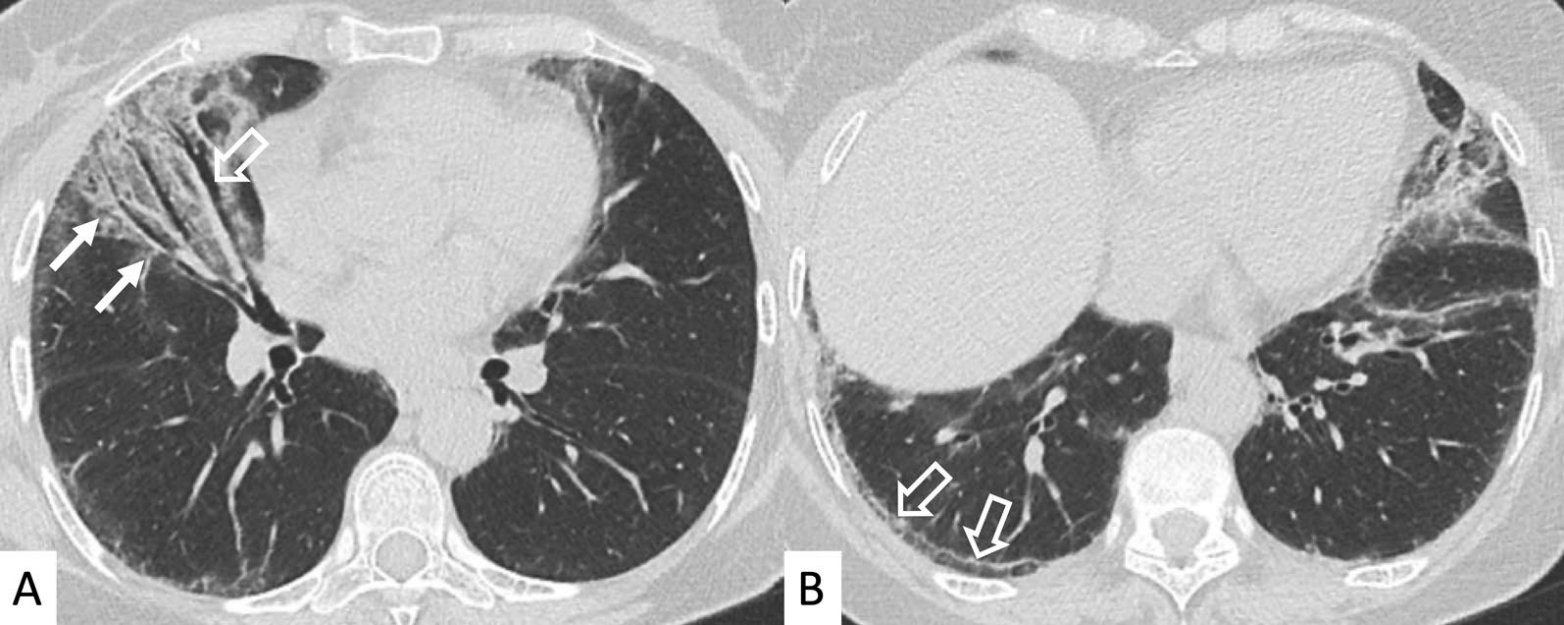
NSIP pattern in a patient with PM. HRCT features include reticulations superimposed on ground-glass opacifications, as clearly depicted in the middle lobe (white arrows); traction bronchiectasis (empty white arrow) can also be appreciated in the same area. Subpleural reticulations are also evident in B, in the basal part of the right lung (empty white arrows)
